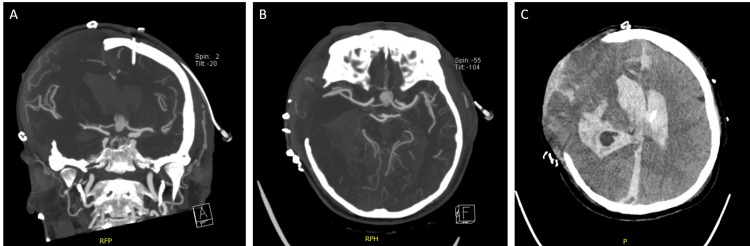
Brain computed tomography taken 12 hours after decompressive craniectomy A) and B) show coronal and axial views of a cerebral computed tomography angiography, respectively, the aneurysm associated with the anterior communicating artery, significant cerebral edema, and a right-sided craniectomy; C) Axial view shows the subarachnoid hemorrhage, a large hemoventricle, hypodensity, and significant cerebral edema and herniation at the site of the right craniectomy, with a ventricular catheter positioned in the left lateral ventricle.
